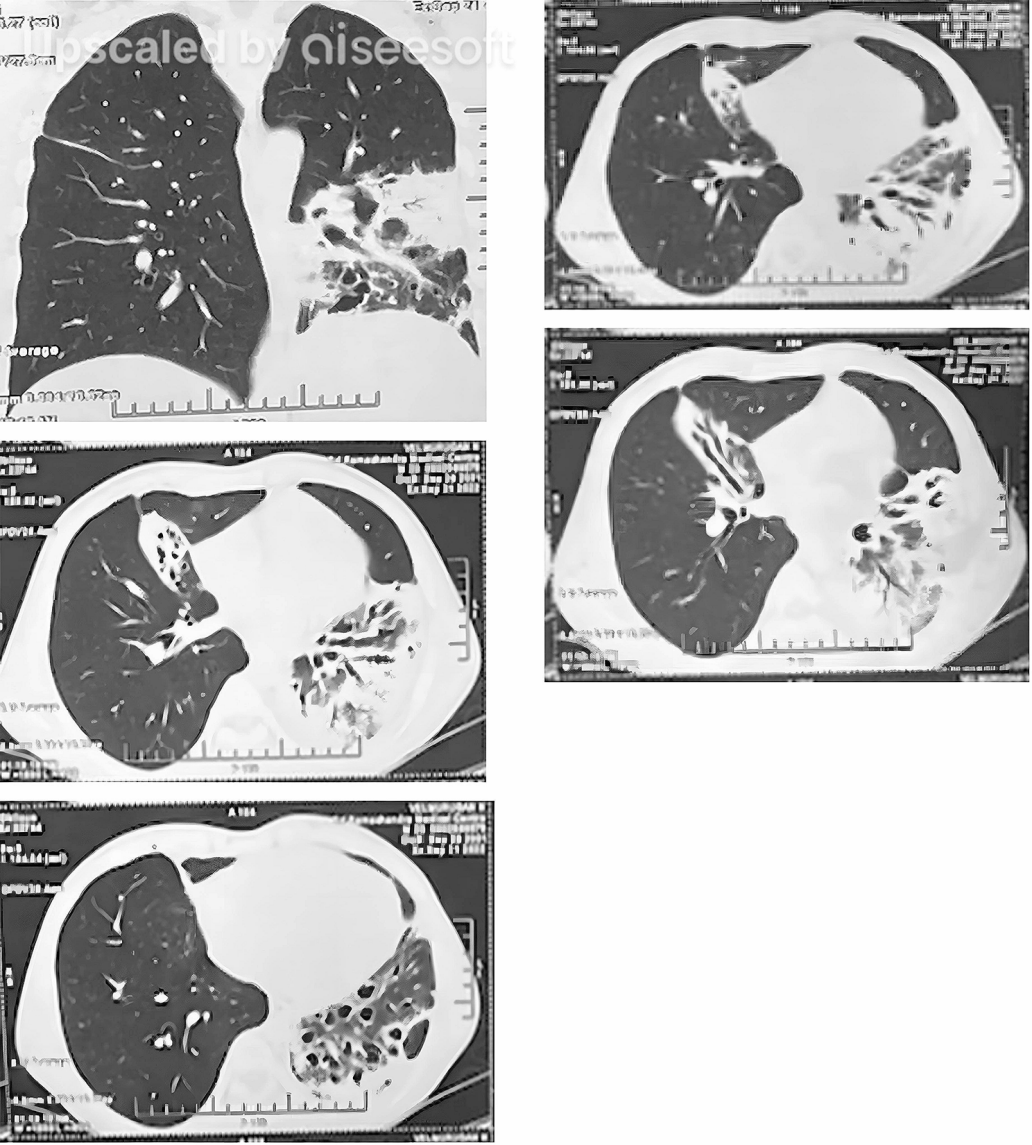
a to e: CT scan of the chest revealed varicose bronchiectasis with surrounding fibrosis and architectural distortion in the lateral segment of the right middle lobe and left lower lobe; patchy areas of consolidation with surrounding ground glass opacities in the lateral segment of the right middle lobe, posterior segment of left upper lobe and left lower lobe; few sub centrilobular nodules in the posteromedial segment of right lower lobe; mediastinal lymphadenopathy
